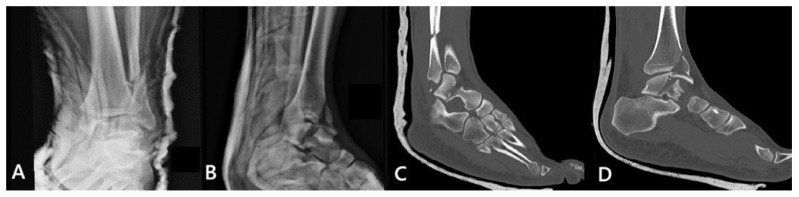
(A) Anteroposterior and (B) lateral preoperative X-ray views of the ankle, showing a talar body fracture combined with an ipsilateral bimalleolar fracture. Sagittal planes in the CT scan of the ankle showing the talar body fracture along with (C) the lateral malleolus fracture and (D) the medial malleolus fracture, respectively.
